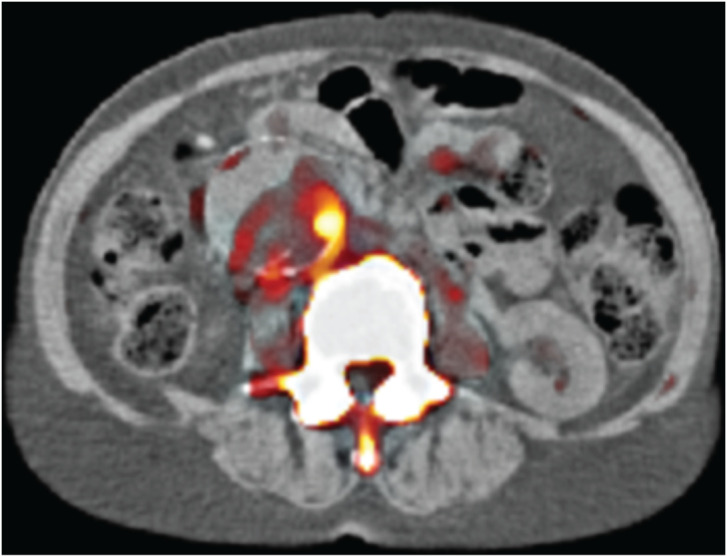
[^18^F]NaF PET image of a patient with abdominal aortic aneurysm as reconstructed with a synergistic algorithm using the CT image for guidance [7]. (Online version in colour.)
